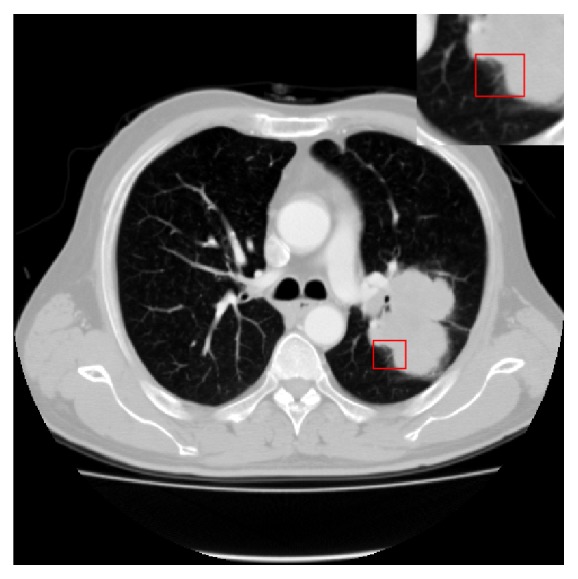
An example of annotated lobulation CT image, where the red rectangle indicates the lobulation region annotated by the radiologist.
